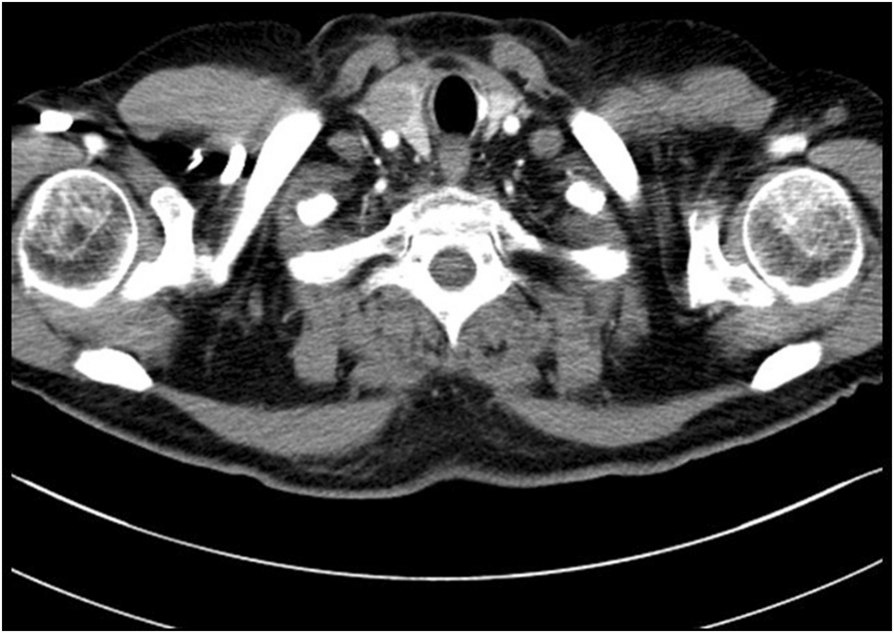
Computed tomography image of the thyroid gland and right thyroid nodule
